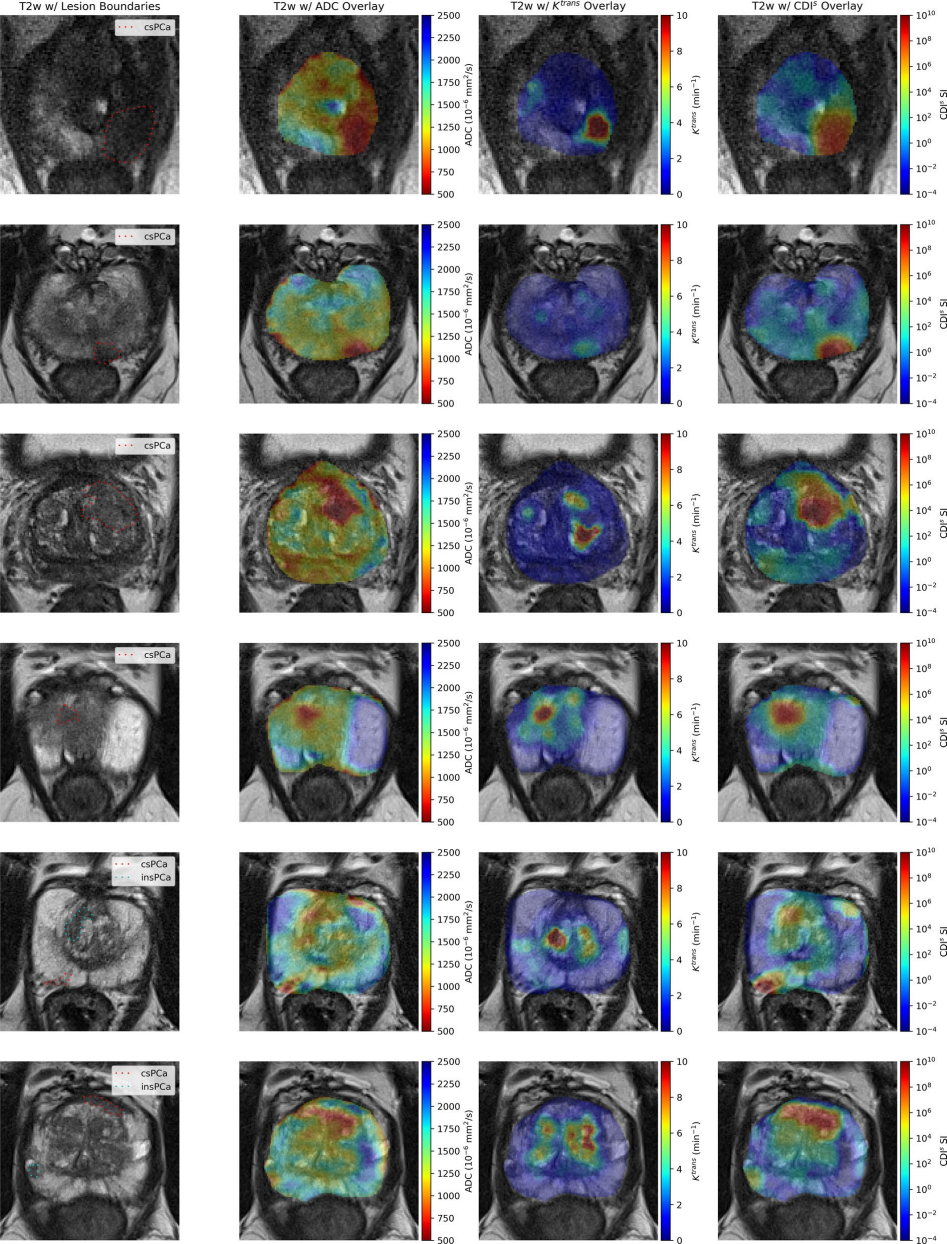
T2w images with overlays of lesion boundaries, DWI-derived ADC, DCE-derived $$K^{trans}$$, and CDI$$^s$$ for six patient cases. (a, b) Two patients with csPCa in the PZ. (c, d) Two patients with csPCa in the TZ. (e) A patient with csPCa in the PZ and insPCa in the TZ. (f) A patient with csPCa in the AS and insPCa in the PZ.
